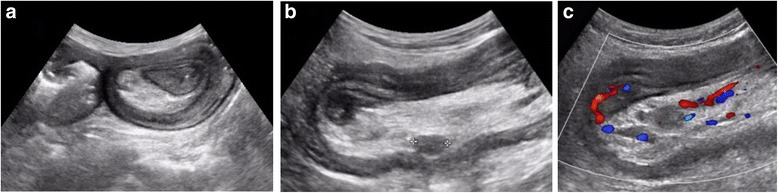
Ultrasonography demonstrated an ileoileal intussusception with the classic “target” sign in the transverse view (a) and showed the typical “pseudo-kidney” sign in the longitudinal view with mesenteric lymph nodes within (b). The color Doppler highlighted the mesenteric vessels within the intestinal lumen (c)
